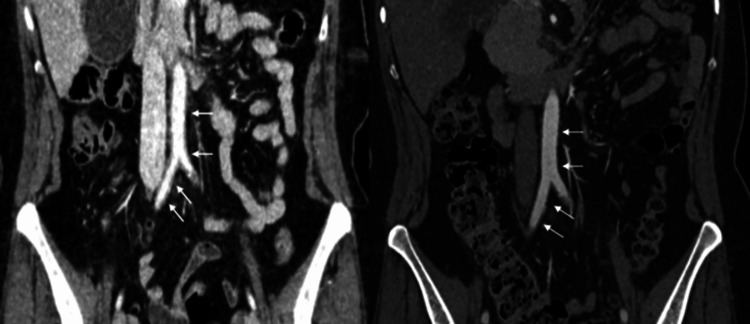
Contrast-enhanced computed tomography (venous phase) of the aortoiliac axis before (A) and after (B) treatment. Coronal image from the pre-treatment study (A) demonstrating laminar, hypodense, and non-stenotic thickening of the aortic wall, consistent with periaortitis, involving the infrarenal abdominal aorta and the common iliac arteries (white arrows). Coronal image from the post-treatment study (B), obtained at the same anatomical levels, showing complete resolution of the wall thickening (white arrows).
